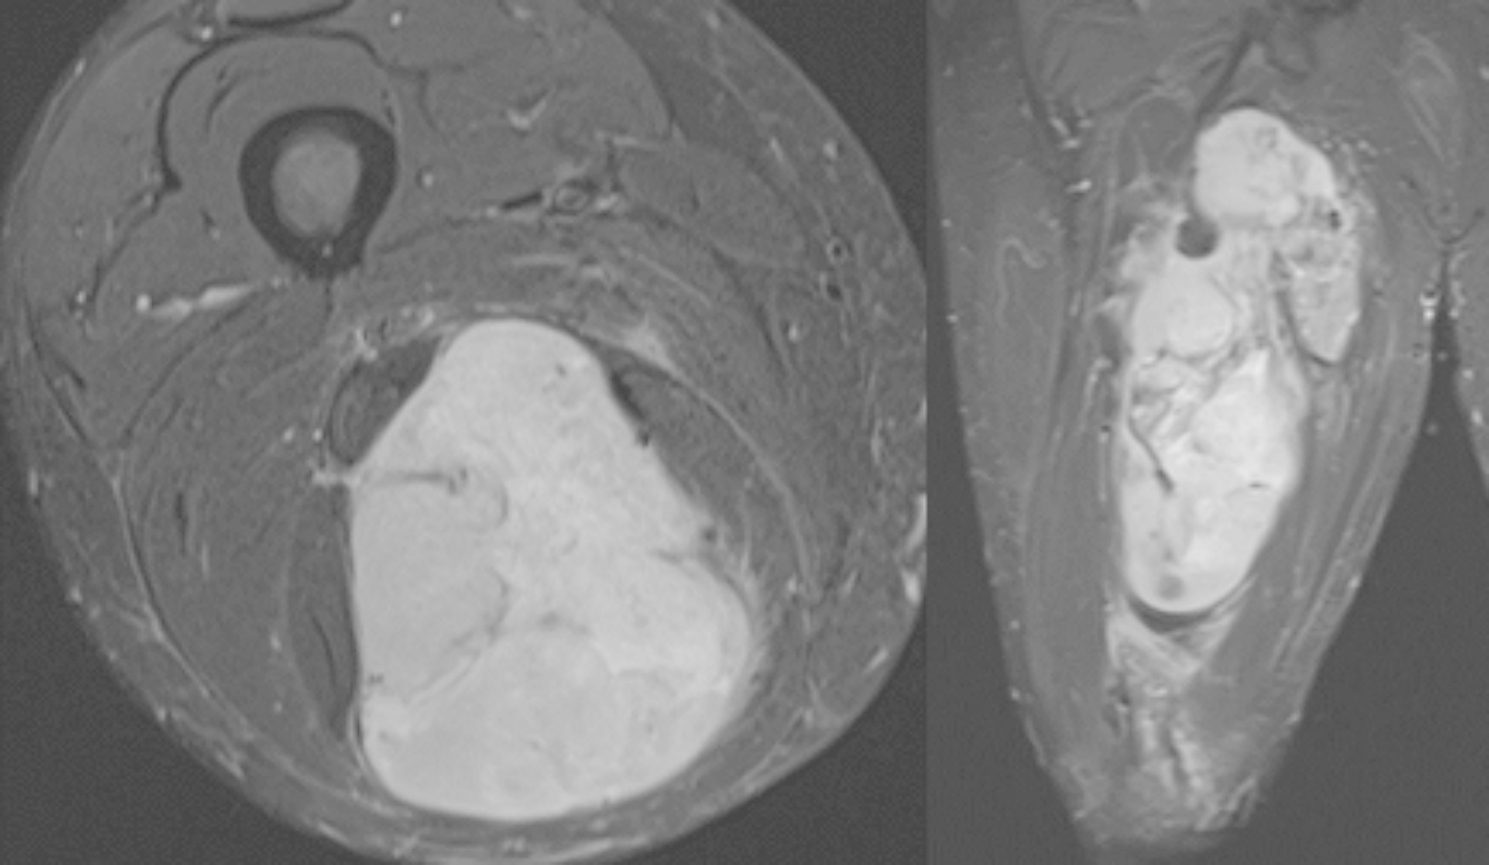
Preoperative T2-weighted MRI (left: axial; right: coronal)
